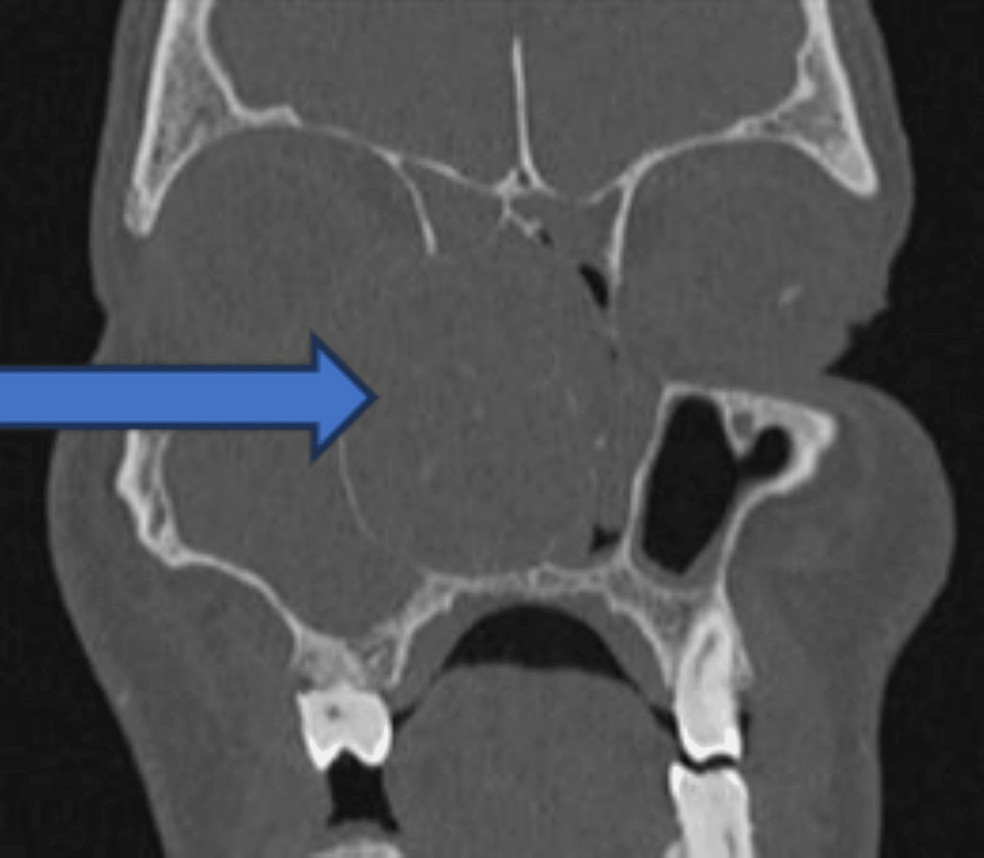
CT scan displaying a sizable, well-defined, smooth-walled lobulated soft tissue lesion centered in the right nasal cavity (solid arrow), measuring 5.6 x 4 x 4.8 cm, resulting in its expansion and protruding into the remaining right maxillary sinus. Erosion of the nasal septum with extension into the left nasal cavity is observed, reaching up to the osteomeatal complex. Superior extension encompasses both the anterior and posterior ethmoid sinuses and the floor of the bilateral olfactory fossa, with the cribriform plate not visualized. Moreover, a superolateral extension infiltrates the medial extraconal compartment of the right orbit, abutting the right medial rectus and superior oblique muscles
